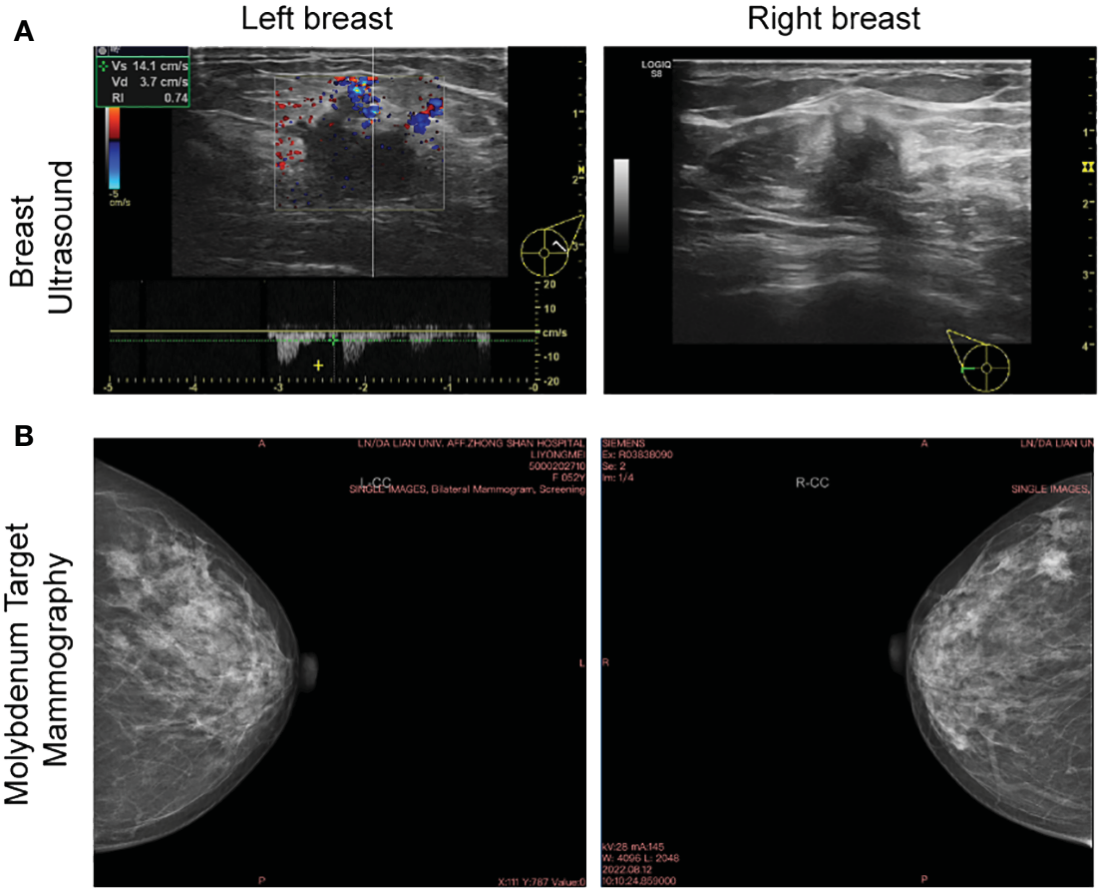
Breast ultrasound and molybdenum target of bilateral breast. (A) The baseline breast ultrasound performed on Aug. 12, 2022 showed solid masses in bilateral breast. (B) Molybdenum target performed on the same day showed nodule in right breast and bilateral mammary gland hyperplasia with benign calcification of the left breast.
